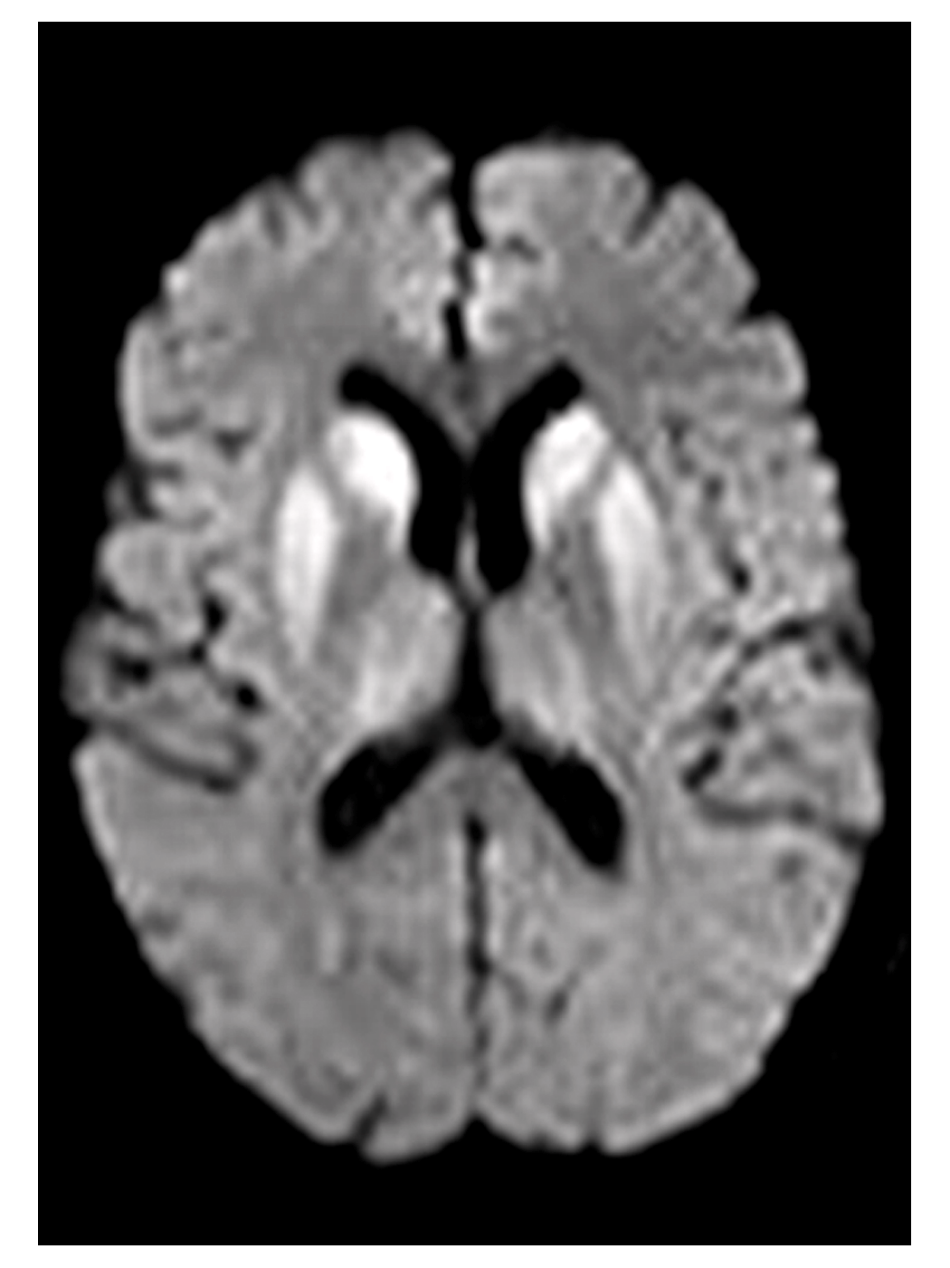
Magnetic resonance imaging of sporadic Creutzfeldt-Jakob disease. Diffusion-weighted image at the level of the basal ganglia demonstrates marked symmetrical hyperintensity in the caudate head and putamen with less marked affection of the thalami. Image courtesy of David Summers, Western General Hospital, Edinburgh, UK.
